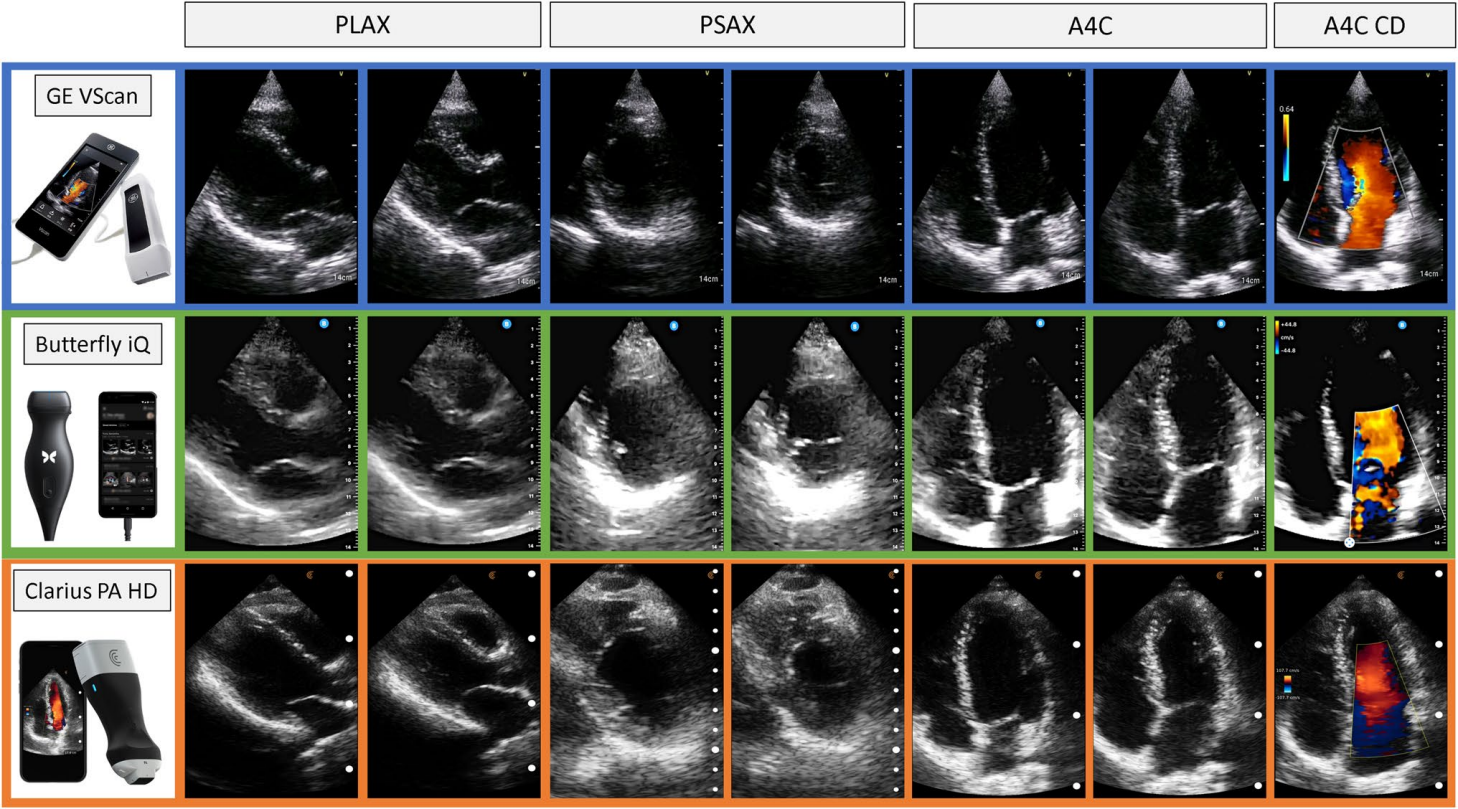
Comparison of main handheld echocardiographic systems available. *PLAX* parasternal long axis, *PSAX* parasternal short axis, *A4C* apical 4-chamber, *CD* colour Doppler
